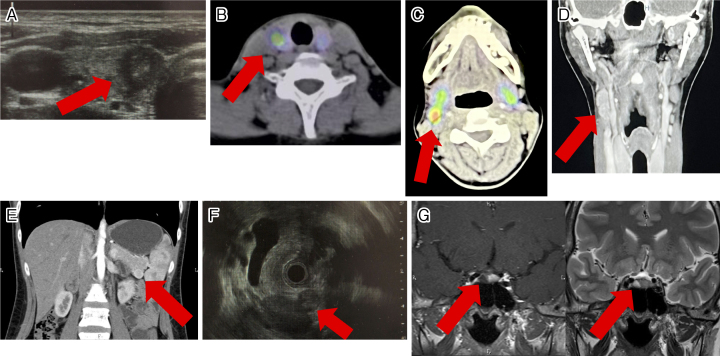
Diagnosis of thyroid tumor and MEN1 by imaging. (A) Ultrasonography of the thyroid gland. (B) ^99m^Tc-MIBI scintigraphy of parathyroid glands. (C) ^99m^Tc-MIBI scintigraphy of the submental region. (D) CT scan of the neck. (E) CT scan of the pancreas. (F) Endoscopic ultrasonography of the pancreas. (G) Enhanced MRI of the pituitary gland. T1 and T2 weighted images (left and right, respectively). Red arrows indicate sites of lesions.
